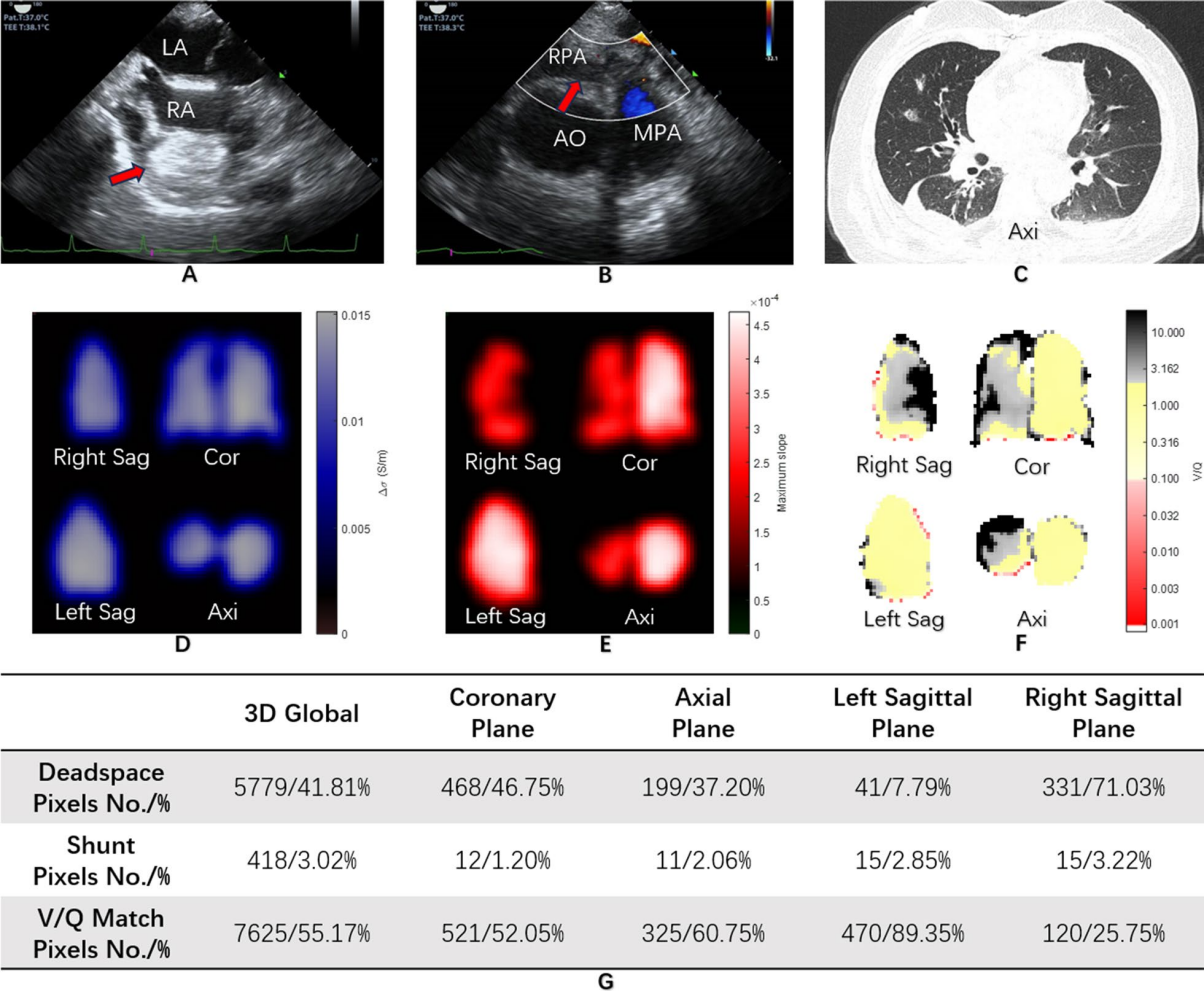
Transesophageal echocardiography (TEE), computed tomography (CT), and saline bolus-based 3D electrical impedance tomography (EIT) results. The EIT measurement system adopted alternating currents with a rms of 2 mA and a frequency of 20 kHz. Voltages were measured at a rate of 20 frames per second. Details of the injection-measurement pattern can be found in [2] (IV-A). The image reconstruction algorithm was run on one NVIDIA Tesla V100 GPU card and the image was produced at a rate of 0.125 frames per second. A TEE image of the mid-esophageal bicaval view. The red arrow indicates a massive embolism in the right atrium. B TEE image of the mid-esophageal ascending aortic short-axis view. The red arrow demonstrates that the color Doppler found no blood perfusion signal in the right pulmonary artery. C Chest CT image indicated that the lung was almost normal. D Lung ventilation image of EIT in coronary, sagittal, and axial planes with low-ventilated regions marked in dark blue and high-ventilated regions in light grey. E Lung perfusion image of EIT in coronary, sagittal, and axial planes. Regions with low perfusion are displayed in dark red and high perfusion in white. F Ventilation/perfusion (V/Q) image of EIT in coronary, sagittal, and axial planes demonstrating the regional V/Q distribution. Regions of dead space (high ventilation and low perfusion) are shown in dark gray. Shunt regions (low ventilation and high perfusion) are marked in red. Areas of matched V/Q ratios are presented in yellow. The cardiac output (2.5 L/min) and minute volume (6 L) were included in the V/Q calculation according to François Perier’s study [3]. G The percentage of deadspace, shunt, and V/Q match pixels in 3D global and different planes. *LA* Left atrium, *RA* Right atrium, *RPA* Right pulmonary artery, *AO* Aorta, *MPA* Main pulmonary artery, *Axi* Axial plane, *Cor* Coronary plane, *Right Sag* Right sagittal plane, *Left Sag* Left sagittal plane
